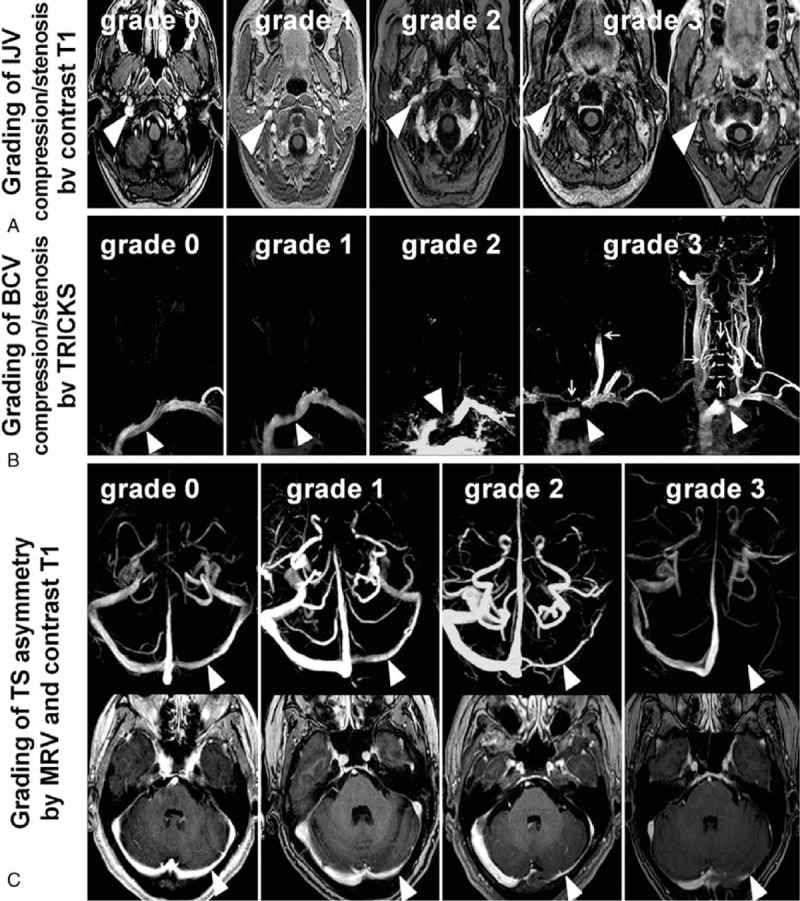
Grading of compression/stenosis using MR imaging. (A) Grading of IJV compression/stenosis by contrast T1: grade 0: normal round or ovoid; grade 1: mild flattening; grade 2: moderate flattening; grade 3: severe flattening, pinpoint or not visualized. (B) Grading of left BCV compression/stenosis by TRICKS: grade 0: normal (arrowhead); grade 1: BCV with mild filling defect by the aortic compression (arrowhead); grade 2: left BCV interrupted at the aortic arch (arrowhead) with filling defect, but without collateral; grade 3: left BCV compression/occlusion (arrowhead) with different types of venous collaterals filling and reflux: venous flow drains across the midline into the right IJV through the anterior cervical veins from left subclavian vein (vertical arrow); IJV reflux (horizontal arrow), the contrast medium injected from the left subclavian vein appears retrograde into the left IJV; Presence of collaterals of vertebral venous system, from the left subclavian vein draining directly through intrarachidian anastomoses to contralateral side at different levels (arrows). (C) Grading of TS asymmetry by MRV: grade 0: symmetrical TS; grade 1: TS asymmetry ≤50%; grade 2: TS asymmetry >50%; and grade 3: aplasia or signal absent (arrowhead pointing out locations for comparison). Grading of TS asymmetry by contrast T1: grade 0: symmetrical TS; grade 1: TS asymmetry ≤50%; grade 2: TS asymmetry >50%; grade 3: aplasia or signal absent (arrowhead pointing out locations for comparison).
